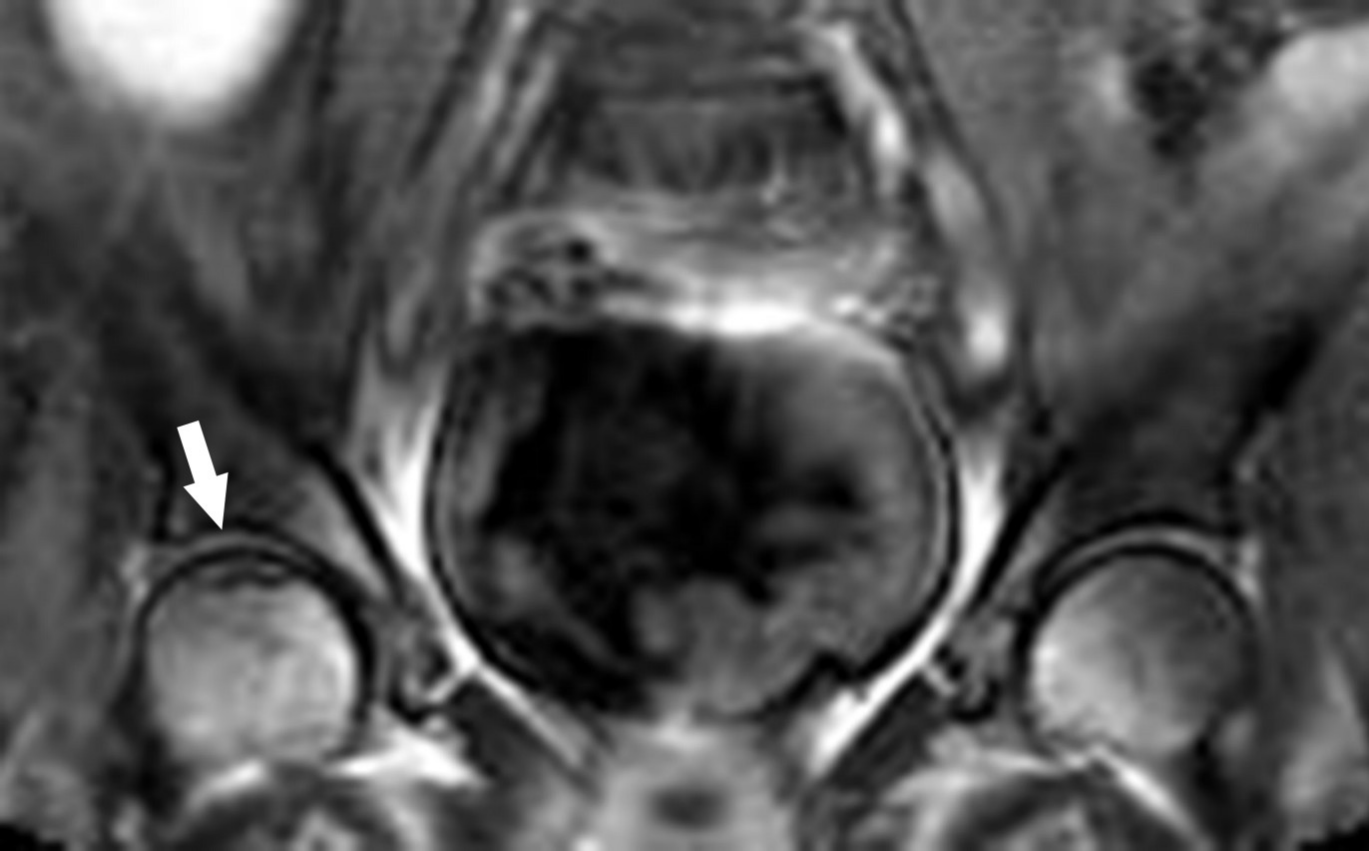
Avascular necrosis of the femoral head on the scout image. Early-stage of avascular necrosis (arrow) of the right femoral head, which is not included in the routine lumbar spine MRI, is incidentally detected on the coronal plane of the scout image.
